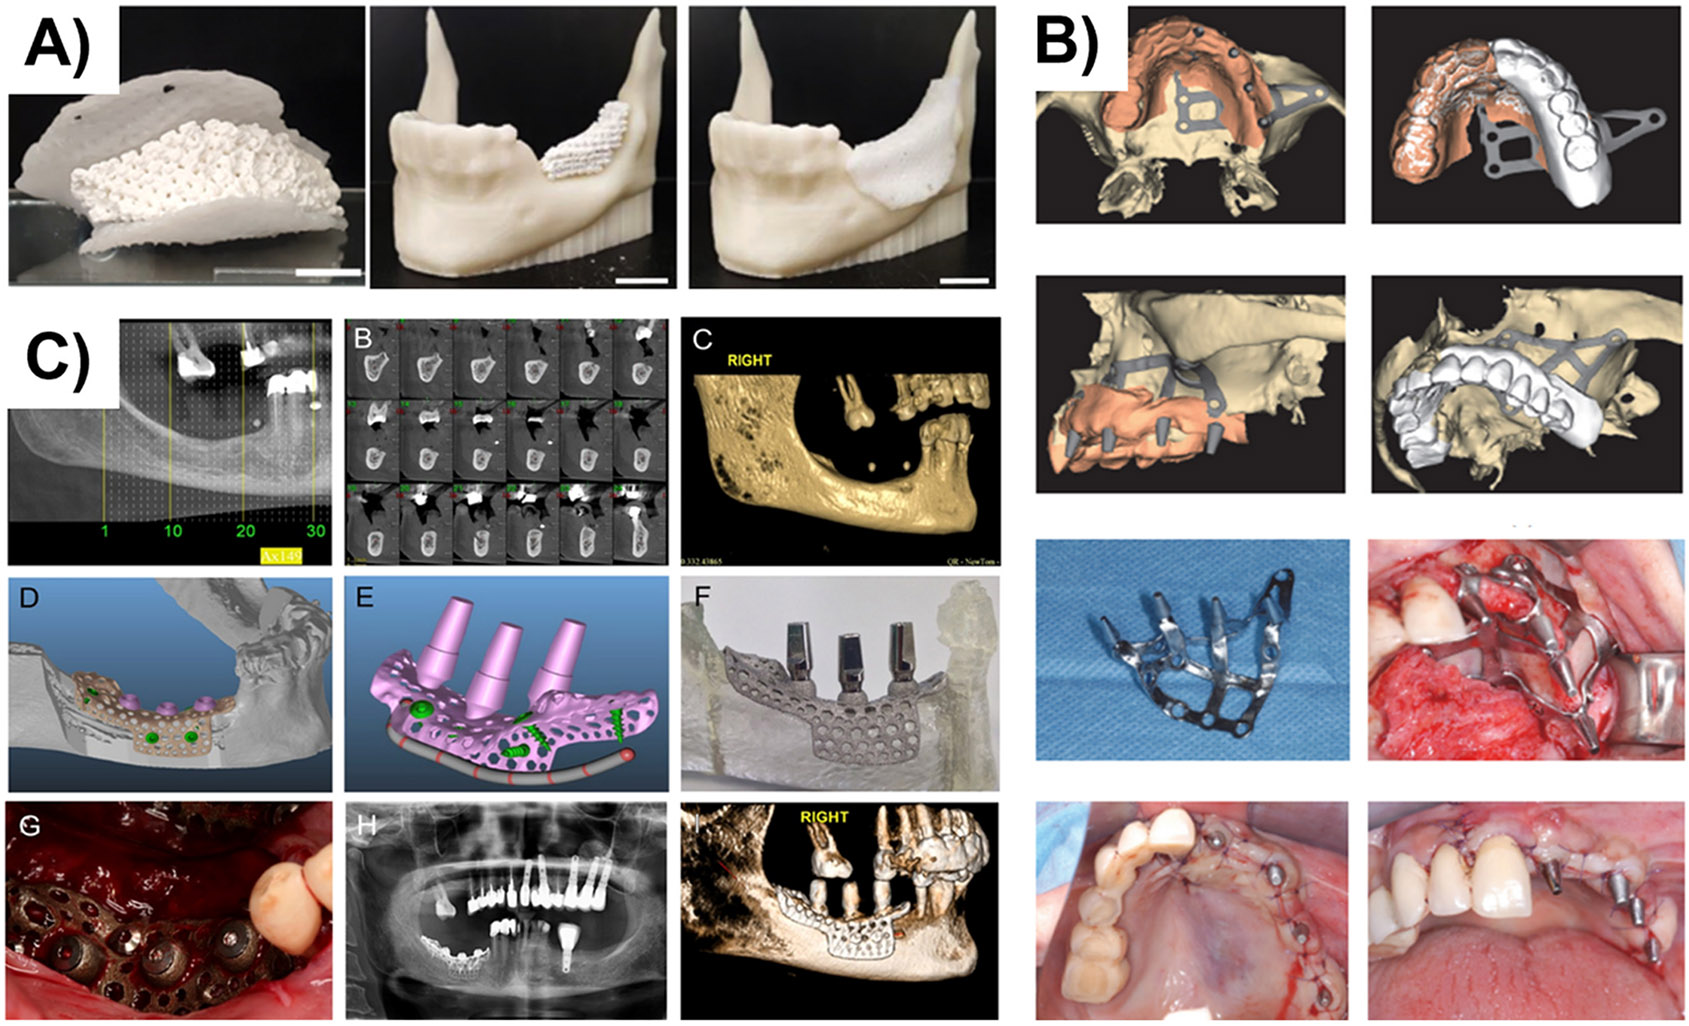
A) Assembly of the osteoconductive 3D printed porous TCP/HA fitted into its PCL cover in a model of the mandibular defect (Reprinted/adapted with permission from [90], 2023, Frontiers); B) A patient-specific endosteal implant for enhancing bone regeneration (Reprinted/adapted with permission from [91], 2019, Hindawi); C) customized Ti-6Al-4 V implant fabricated as one object and inserted in the patient, a three month follow up using X-ray observation to evaluate osseointegration and the bone to implant contact (Reprinted/adapted with permission from [92], 2016, Nature).
